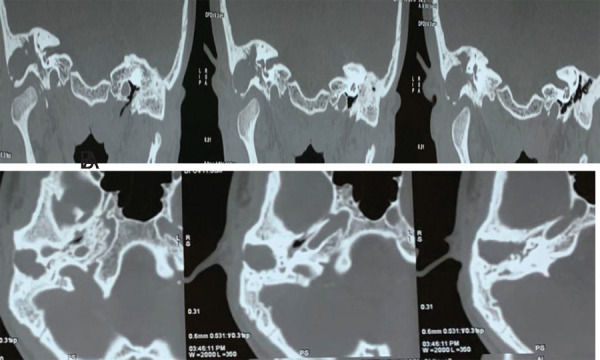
A, B. HRCT of the temporal bone-coronal and axial view.
